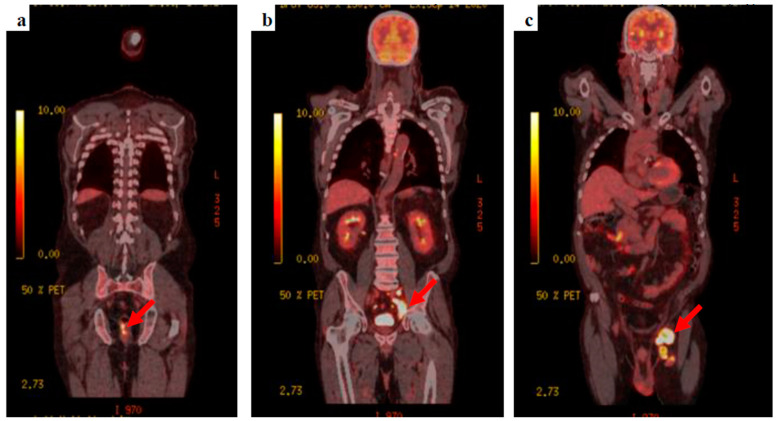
^18^F-FDG PET-CT images (a) the anorectal primary lesion with SUV_max_ 12.2, (b) deep, left external and common iliac nodal uptake, and (c) bulky, extensive, left inguinal lymphadenopathy.
